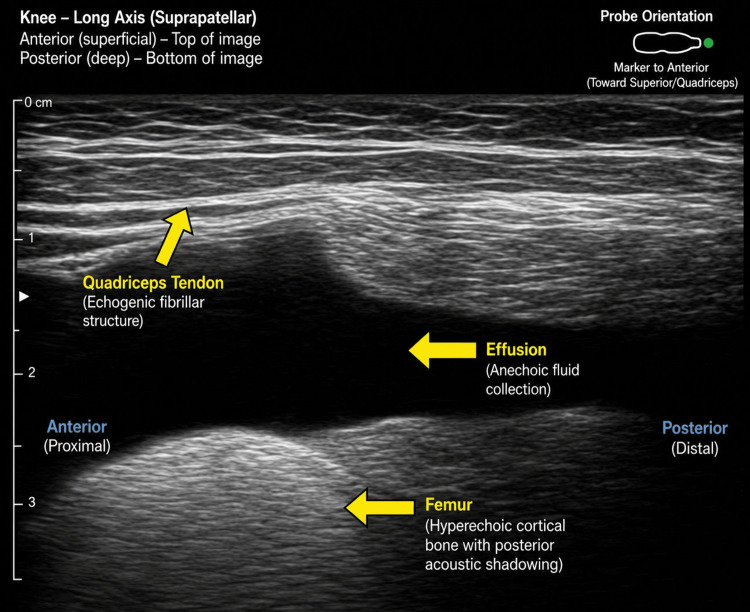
Ultrasound image of the left suprapatellar recess demonstrating anechoic fluid distension consistent with joint effusion.
